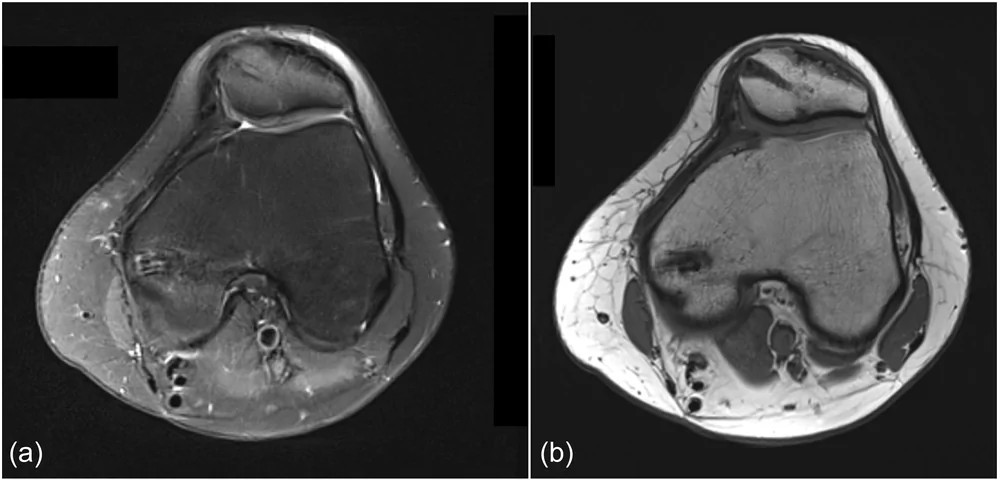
Postoperative magnetic resonance imaging (MRI) 2 years after performing the parachute technique in a right knee after traumatic patellar dislocation with an osteochondral flake fracture. Osteochondral integration is visible on the (a) axial T2‐weighted and (b) intermediate‐weighted turbo spin‐echo (TSE) images.
